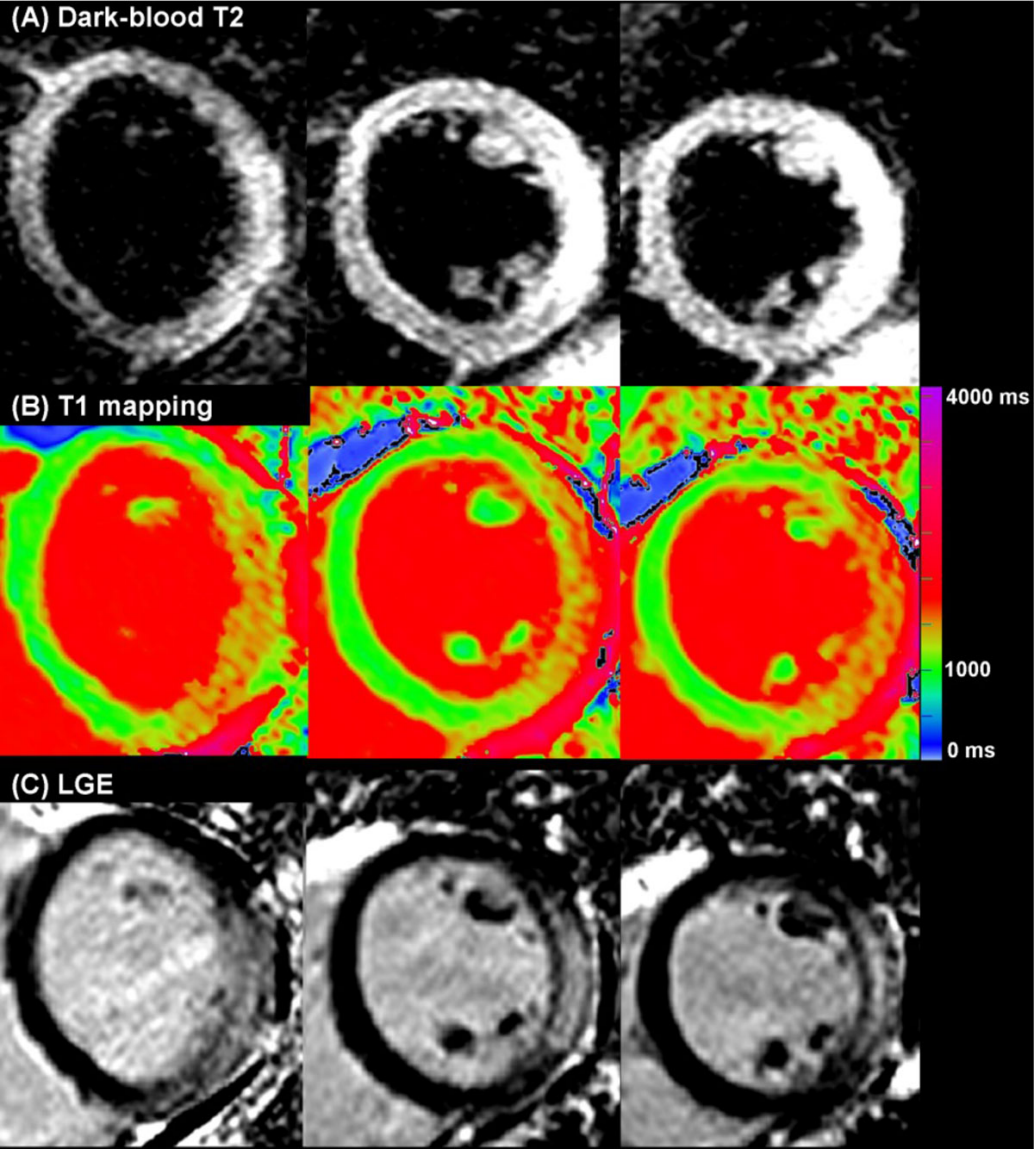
CMR tissue characterization using (A) dark-blood T2-weighted imaging; (B) native T1-mapping (ShMOLLI); (C) late gadolinium enhancement imaging.
